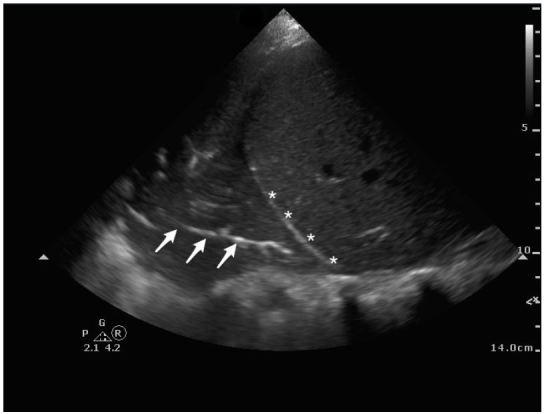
Ultrasound of lung with pneumonia: Linear, bright (hyper-echoic) foci represent air bronchograms (arrows) above the diaphragm (asterisks).
